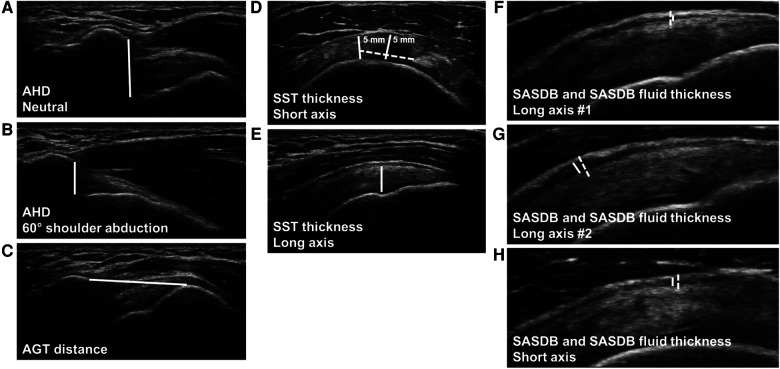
Ultrasound (US) measurements of acromiohumeral distance (AHD), acromion-greater tuberosity (AGT) distance, supraspinatus tendon (SST) thickness, and subacromial-subdeltoid bursa (SASDB) and SASDB fluid thickness. US images were captured in two positions for AHD measurements: (A) in neutral position with the forearm pronated, resting on the ipsilateral thigh, and (B) at 60° active shoulder abduction in the coronal plane. AHD was measured as the shortest tangential distance (line) between the hyperechoic landmarks of the acromion and the superior-most aspect of the humerus. (C) AGT distance was measured as the shortest distance (line) between the lateral edge of the acromion and the apex of the greater tuberosity of the humerus. SST thickness in short axis (D) was obtained as the average of measurements (lines) at two points, 5 mm and 10 mm posterior to the edge of the biceps tendon (dashed lines). SST thickness in long axis (E) was measured at the deepest portion of the superior facet of the greater tuberosity (line). SASDB thickness (dashed lines) was measured by obtaining images in three positions: in the longitudinal plane over the more objective, anterior-most portion of the greater tuberosity (long axis #1, F) and the subjective point of greatest thickness, determined by the rater (long axis #2, G), and in the transverse plane (short axis, H). SASDB fluid (lines) was measured as a hypoechoic line between two layers of peribursal fat in the same three positions (long axis #1 and #2, short axis, F–H).
